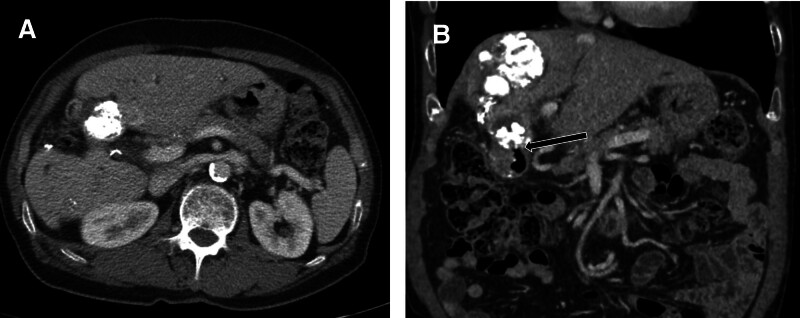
Postoperative CT scans. (A and B) Three weeks after procedure, there was a compact lipiodolized tumor in S4b. A slight protrusion of iodized oil into the duodenal lumen was noted (arrow). CT = computed tomography.
